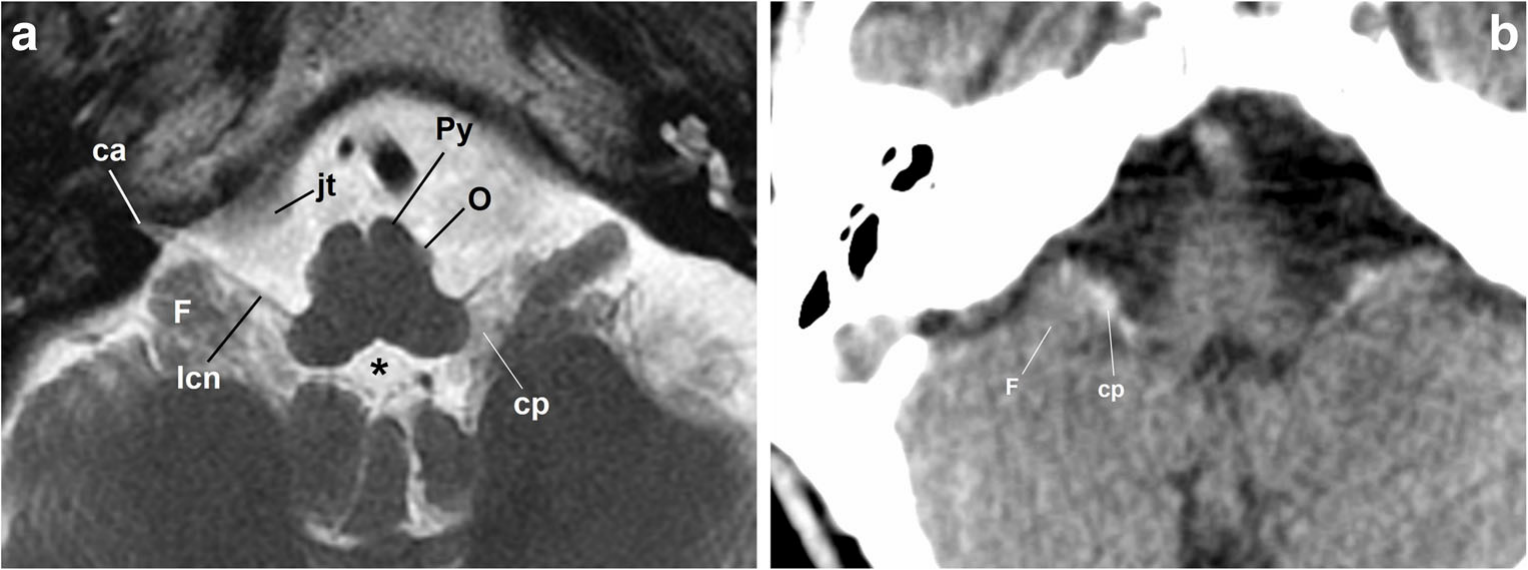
a Axial fast spin-echo T2-weighted brain MRI at the level of the medulla oblongata. The image shows the lower cranial nerves (lcn) passing by the flocculus (F), the choroid plexus (cp) outside the fourth ventricle (*) and the jugular tubercle (jt) as they approach the cochlear aqueduct (ca); O: inferior olivary nucleus; Py: pyramid. b Axial non-enhanced brain CT scan, brain window. The flocculus (F) and the choroid plexus (cp) are reliable references to determine the position of the nearby glossopharyngeal nerve
